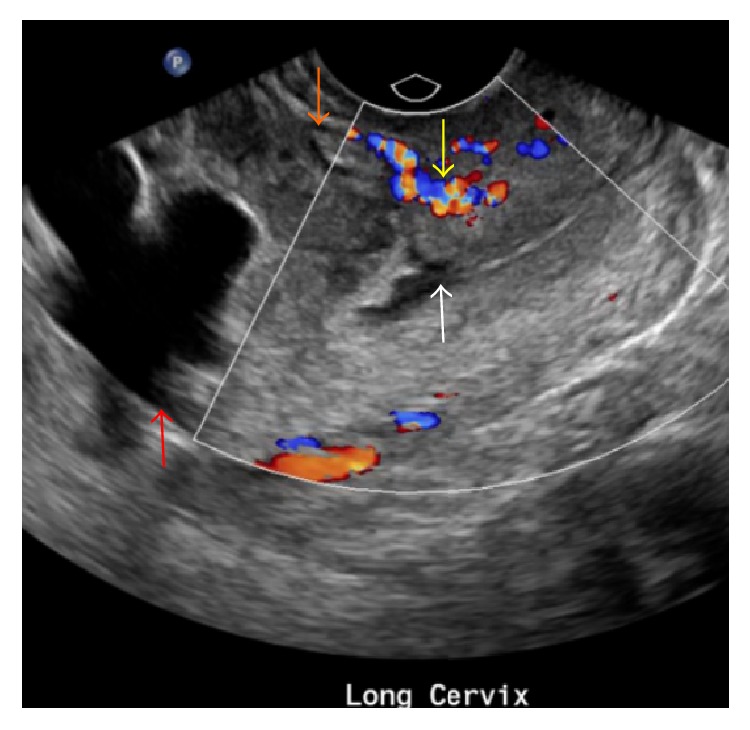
Longitudinal grayscale and color Doppler image of the lower uterine segment and cervix demonstrates endometrial fluid (red arrow) and an irregular, heterogeneously hypoechoic placenta (orange arrow) with blood supply from cervical vessels (yellow arrow) extending into the anterior myometrial wall and over the internal cervical os (white arrow).
